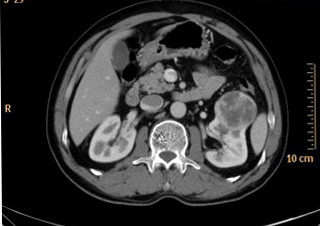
Contrast enhanced abdominal CT scan shows a well-delineated heterogeneous soft-tissue mass with small areas of calcification in the left kidney.
